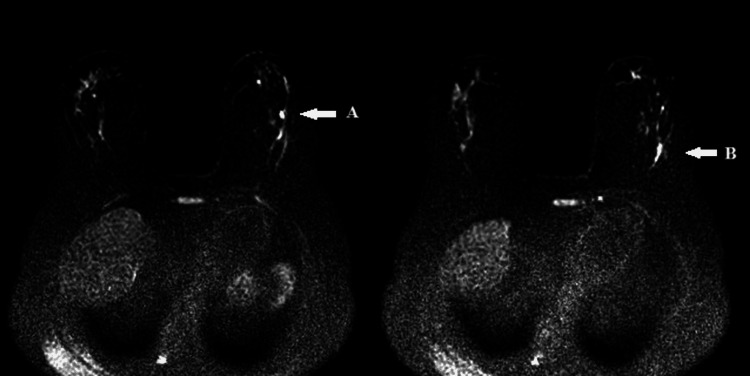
Axial diffusion-weighted breast MRI. Areas of focal enhancement in the left breast (A, B).
